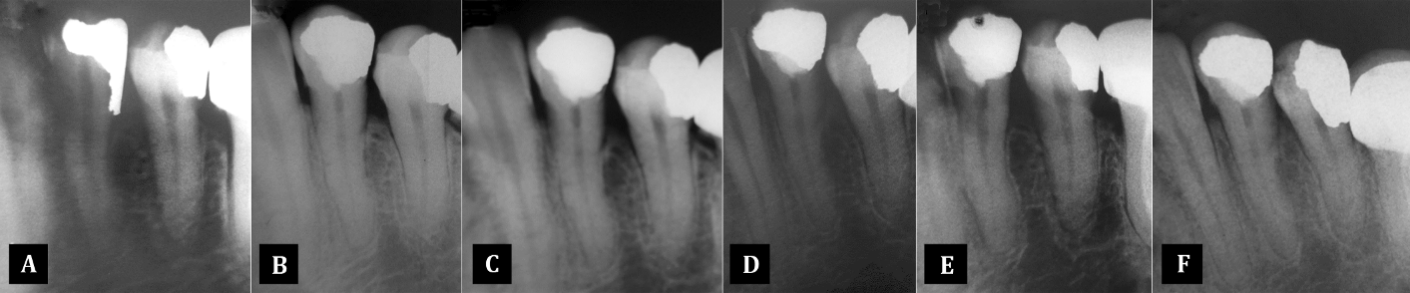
Periapical diagnostic and follow-up radiographs of tooth 34; A) diagnostic radiograph showing a secondary caries under amalgam restoration; B) 3 month after MTA pulpotomy; the periapical structures appear normal and; C-F) normal periapical structures upon radiographic follow up visits at 12, 24, 36 and 42 months, respectively
